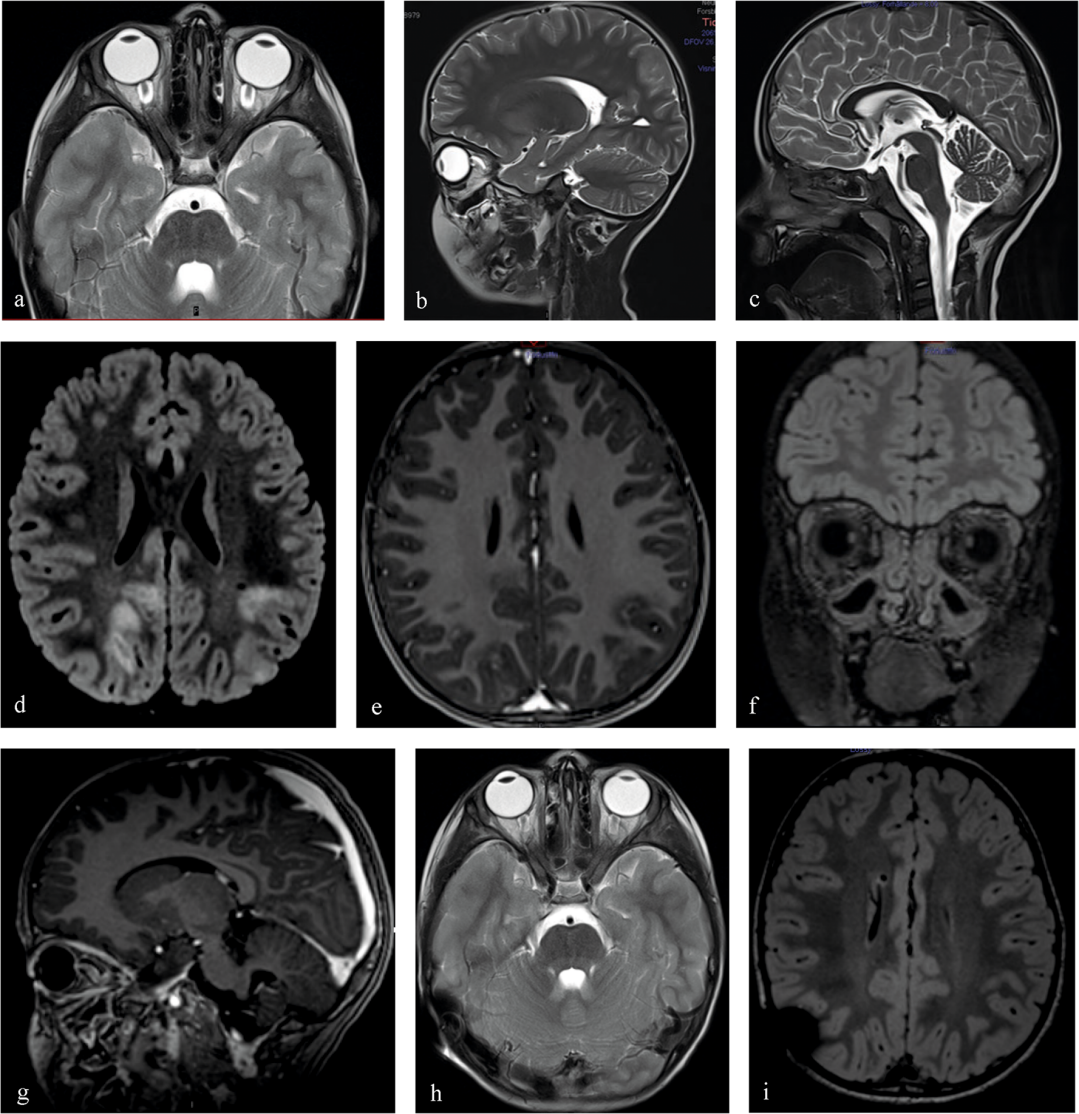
a–c T2-weighted sequences from the initial MRI of the brain, performed during investigation of the headache and nausea, demonstrate findings consistent with IIH. a Transverse T2-weighted image shows significant distention of the perioptic nerve sheaths with flattening of the posterior sclera of both globes; b sagittal T2-weighted image showing same findings and in addition vertical tortuosity of the intraorbital optic nerve; c sagittal T2-weighted image with mild reduction in the height of the hypophysis. d–g MRI sequences obtained at the time of rapid deterioration with impairment of vision and encephalopathy. d Transverse FLAIR image demonstrates multiple bilateral subcortical hyperintense lesions dominating in the parietal and occipital lobes; e transverse T1 gadolinium (Gd) image shows incomplete ring or nodular contrast enhancement in some of the subcortical lesions; f coronal FLAIR image shows bilateral optic nerve head protrusion into the globes; g sagittal T1 Gd image showing protrusion of the optic nerve head into the globes and diffuse contrast enhancement in both optic nerves. h–i: Follow-up MRI 2 months after VP shunt placement and steroid treatment. h T2 transverse image shows regress of distention of perioptic nerve sheaths, posterior globe flattening, and optic nerve head protrusion into the globe; i FLAIR transverse image shows complete regression of the intracerebral signal changes
